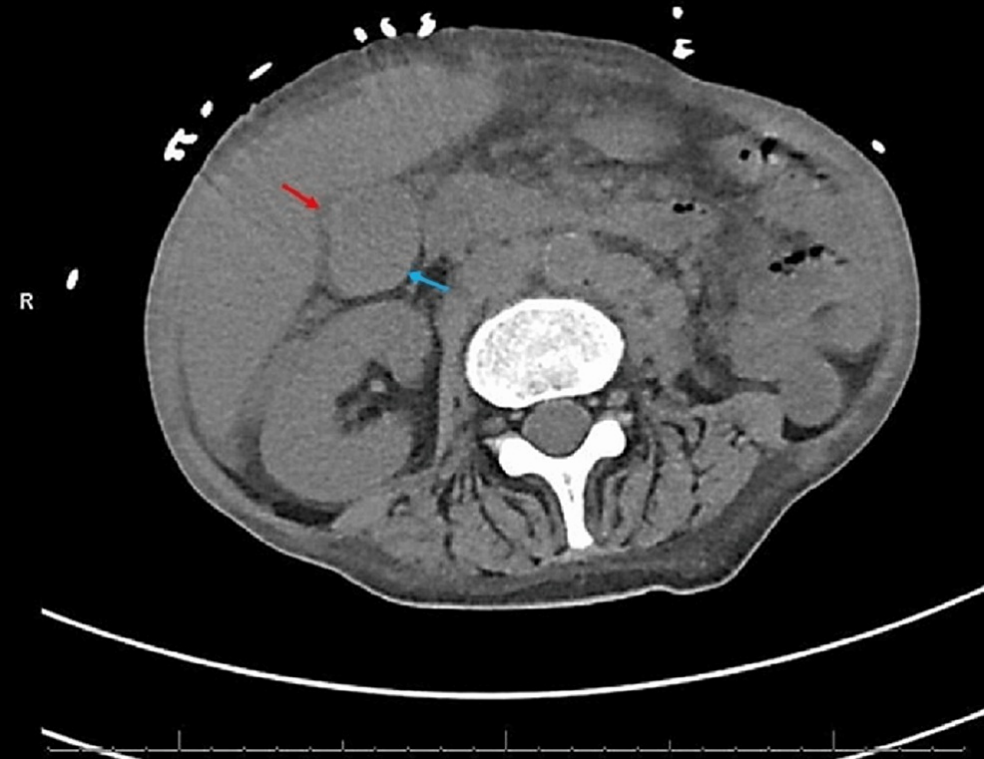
Computed tomography of the abdomen (axial view) showing a distended gallbladder (blue arrow) with a thickened wall and pericholecystic fluid (red arrow).
